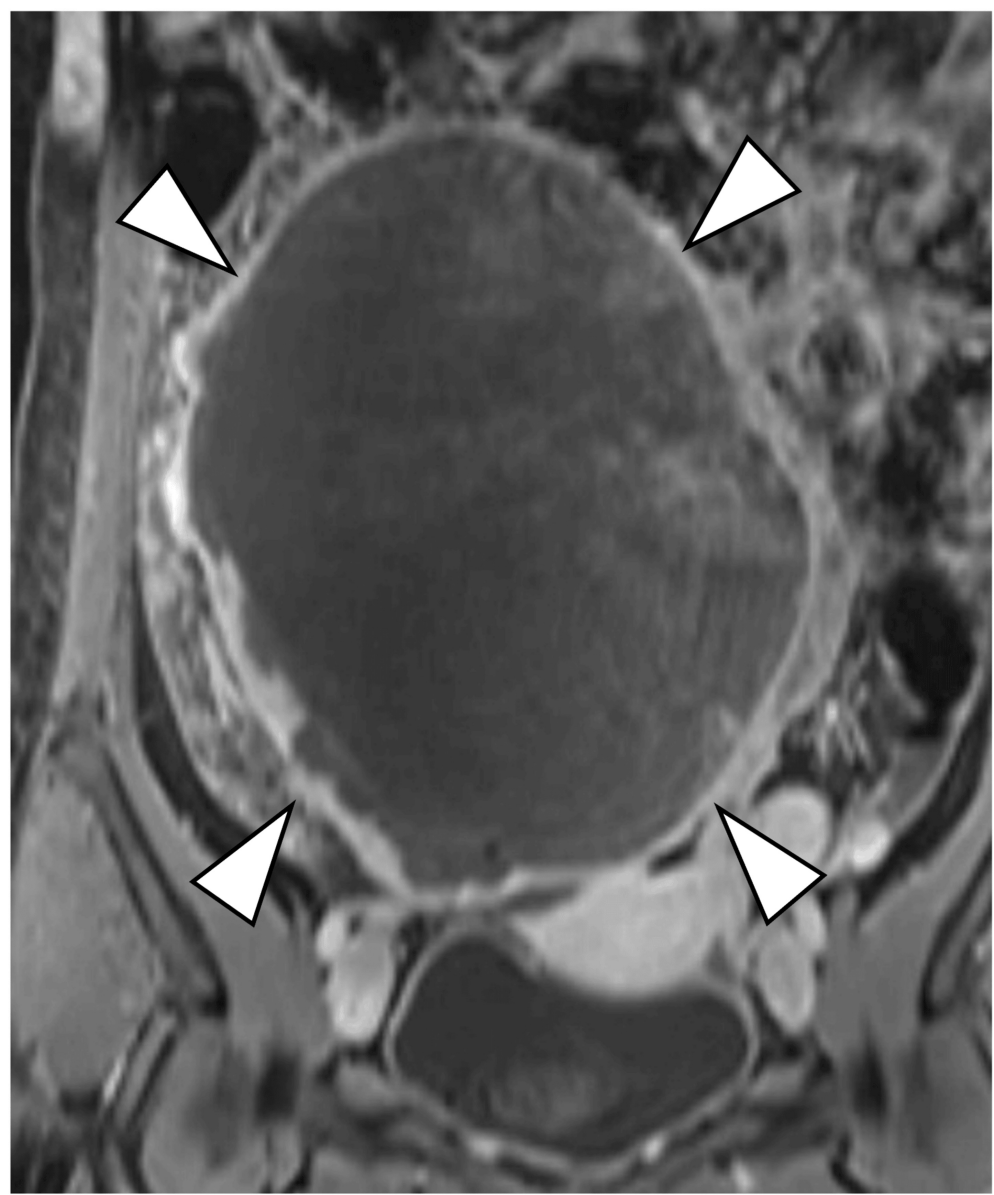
Coronal, contrast-enhanced fat-suppressed T1-weighted Contrast-enhanced fat-suppressed T1-weighted images show that only the capsular structures of the mass are enhanced (arrowheads).
